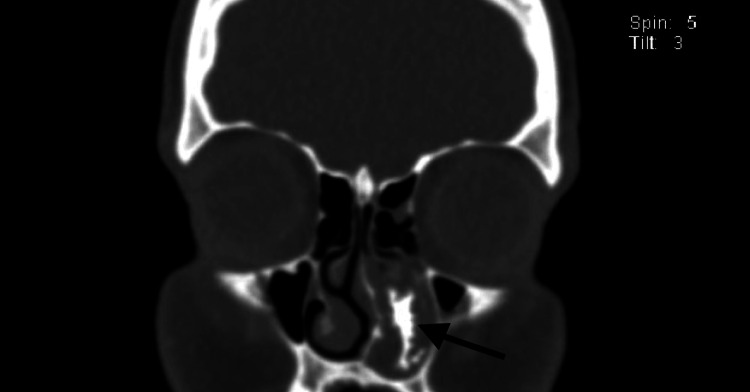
Coronal CT showing calcified iso-dense mass lesion (black arrow) measuring 2.7 × 3.4 cm that is occupying most of the left nasal cavity CT: computed tomography
